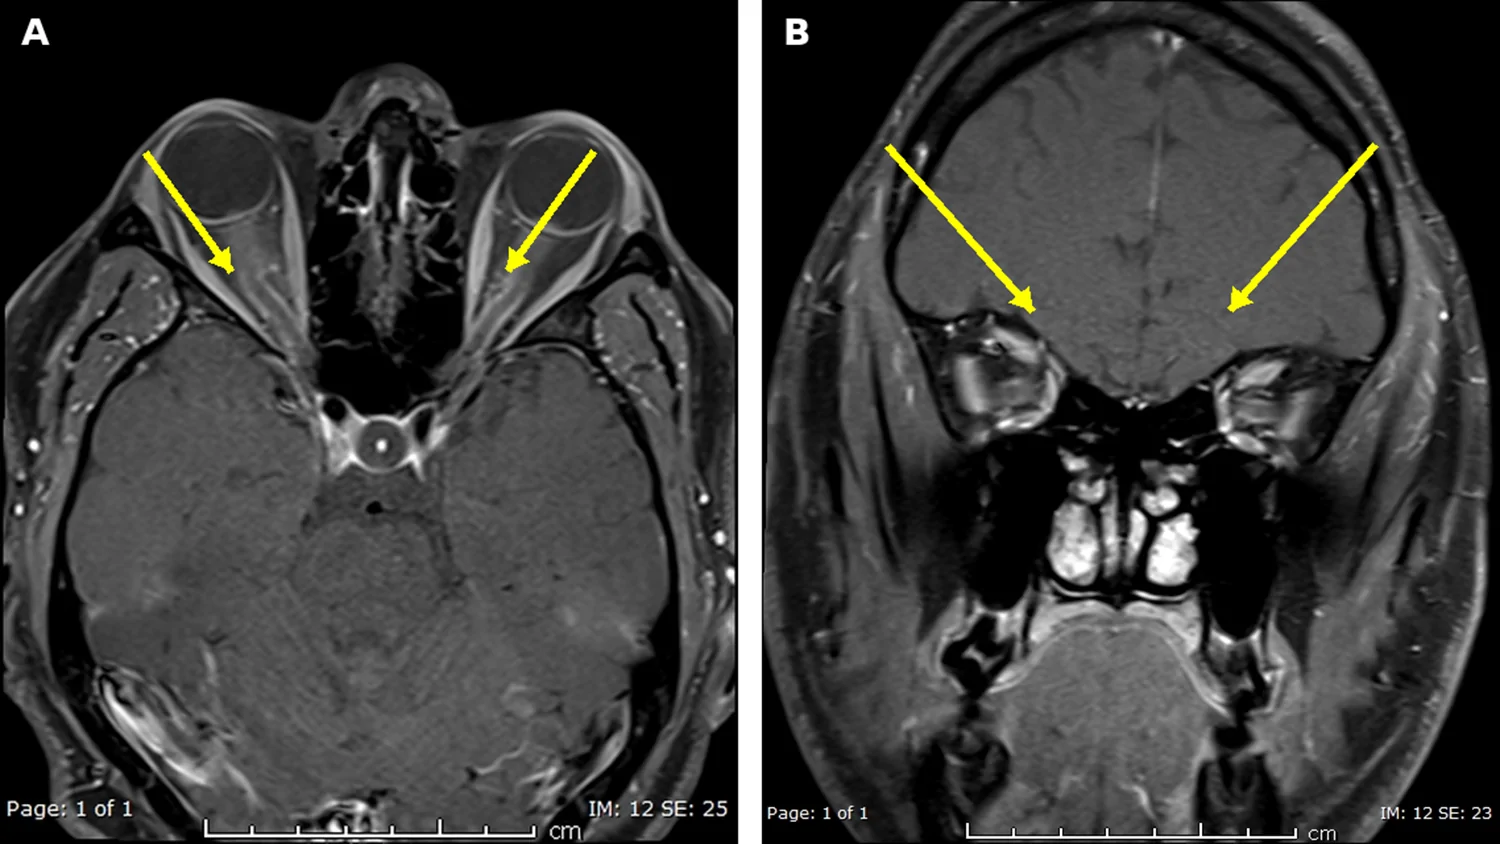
MRI features of bilateral peri-optic neurolymphomatosis in a patient with T-lymphoblastic leukemia/lymphoma. (A) Axial and (B) coronal post-contrast fat-saturated T1-weighted brain MRI images show uniform thickening and enhancement of the peri-optic nerve meningeal sheaths (yellow arrows).
